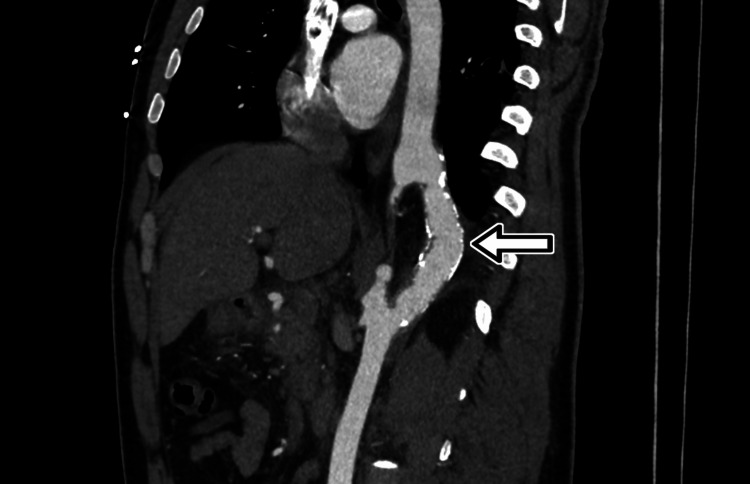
Sagittal CT angiography. The sagittal reformation demonstrates the full course of the patent trunk (arrow).
